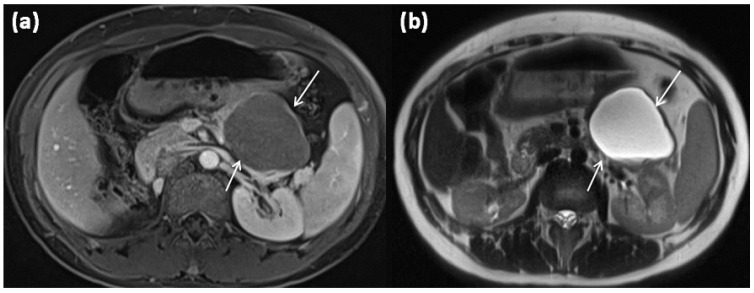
Axial MRI of a 12-year-old female patient with T1w sequence after gadolinium-based contrast administration (a) and T2w sequence (b). The pancreatic body shows a relatively large, sharply demarcated lesion compared to the surrounding parenchyma, which corresponds to a simple ductal cyst (arrows). No septa or nodular proliferations are detectable, nor are environmental reactions or infiltrative growth present.
